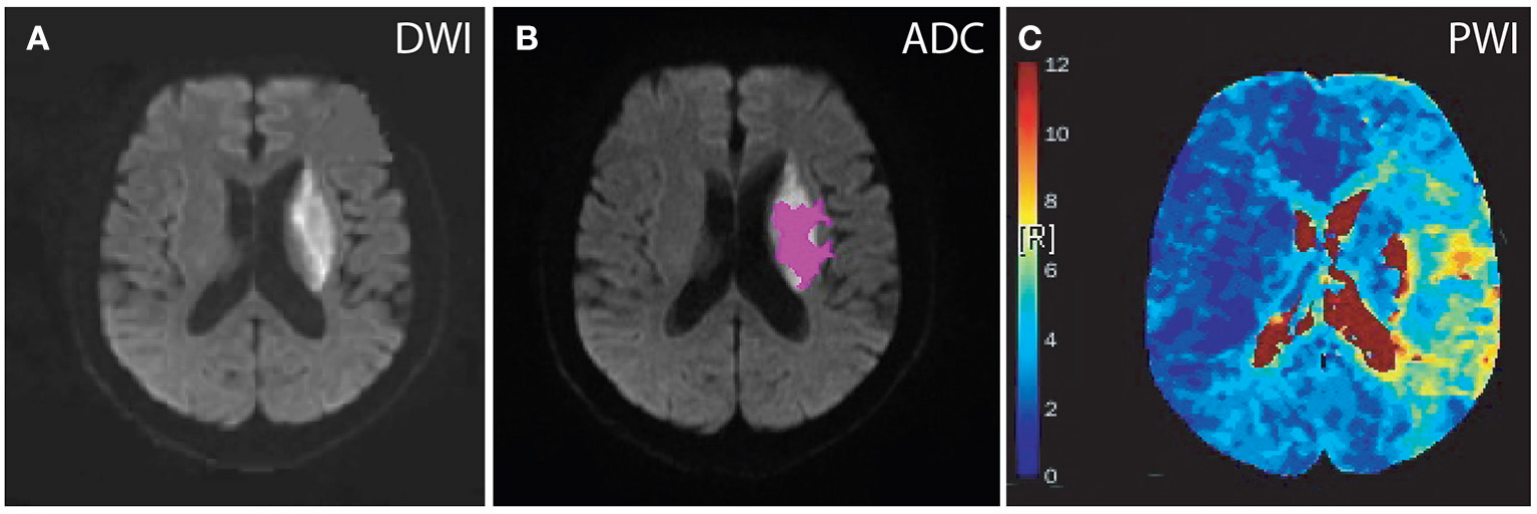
Diffusion and perfusion abnormalities of a patient treated with intravenous tPA. A 63-year-old male who presented with dysarthria and weakness in the right arm, hypertension for more than 3 years, vascular stenosis (≥70%), no previous ischemic stroke, MRI scan was completed 6 days after stroke onset. DWI, diffusion-weighted imaging; PWI, perfusion-weighted imaging; ADC, apparent diffusion coefficient; *T*_*max*_, Time to maximum of the residue function. *T*_*max*_ color scale: 4 s < *T*_*max*_ ≤ 6 s (blue); 6 s < *T*_*max*_ ≤ 8 s (green); 8 s < *T*_*max*_ ≤ 10 s (yellow); 10 s < *T*_*max*_ (red). (A) DWI, lesion volume was 22 ml. (B) ADC, lesion volume was 9 ml. (C) PWI, lesion volumes according to *T*_*max*_ delay were as follows: *T*_*max*_ > 4 s, 132 ml; *T*_*max*_ > 4 s–ADC volume, 123 ml; *T*_*max*_ > 6 s, 22 ml; *T*_*max*_ > 6 s–ADC volume, 13 ml; *T*_*max*_ > 8 s, 4 ml; and *T*_*max*_ > 10 s, 3 ml.
